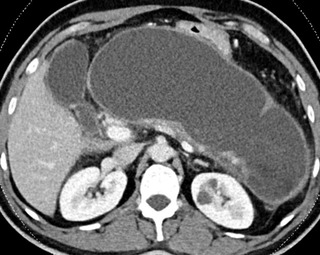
Computed tomography of large walled‐off necrosis prior to endoscopic intervention
